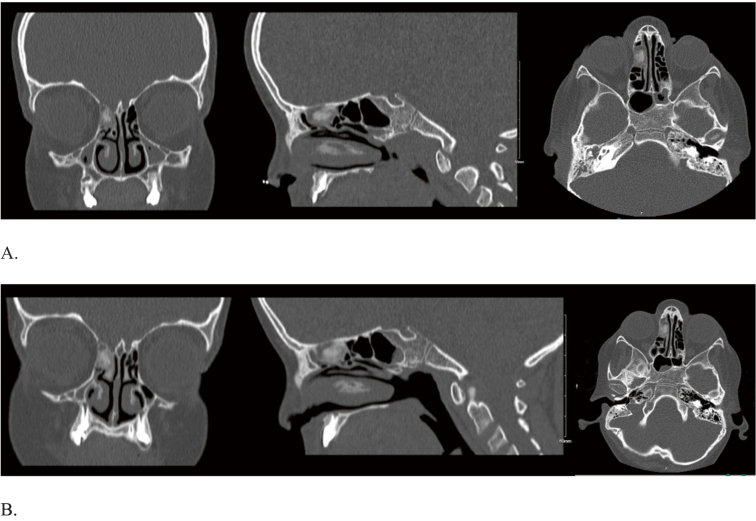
A) Different views of the sinus CT scan at the first presentation showing right anterior ethmoidal sinus complete hyperdense opacification. B) Different views of the second sinus CT after antimicrobial management showing the persistence of the right anterior ethmoidal sinus complete hyperdense opacification.
